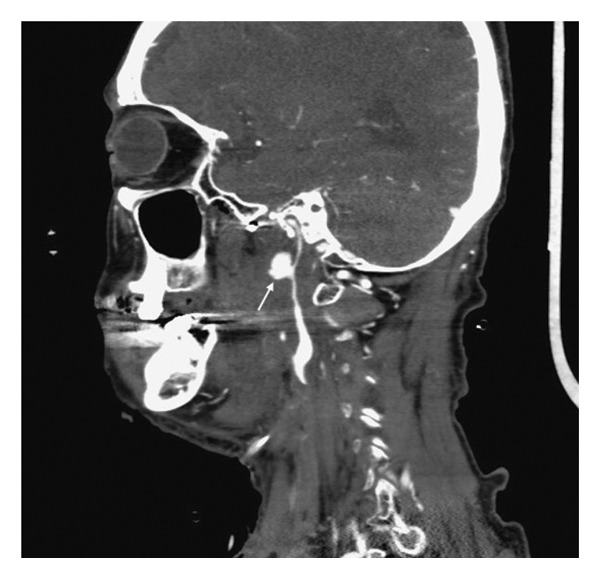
Second episode of carotid blowout syndrome. Computed tomography angiography (CTA) demonstrates a pseudoaneurysm of the left internal carotid artery (ICA) near the skull base (arrow), distal to the previously ligated common carotid artery (CCA).
